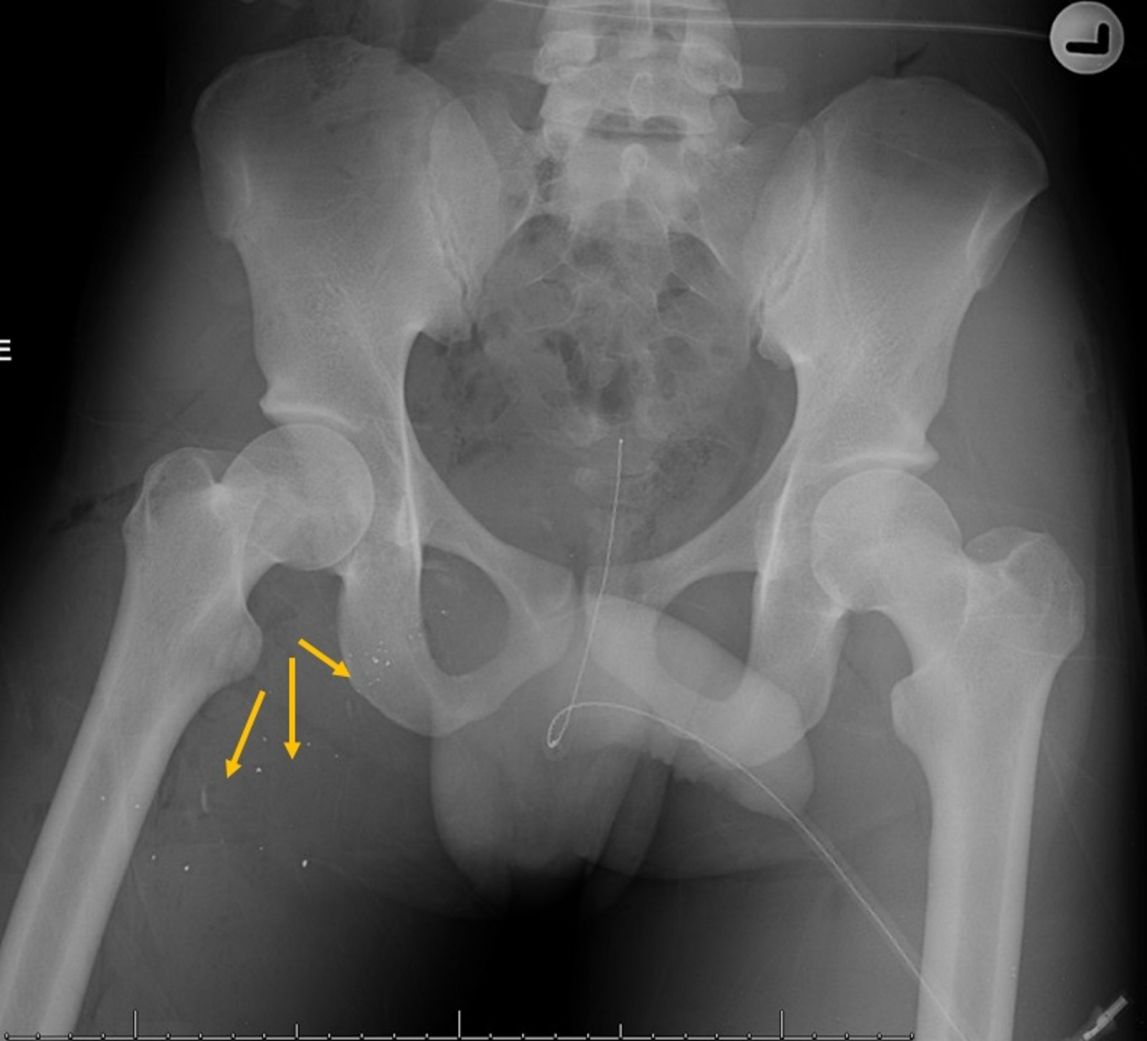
Pelvic X-ray Anterior-posterior (AP) pelvis X-ray demonstrating metallic bullet fragments (arrows) overlying the superior medial right thigh.
